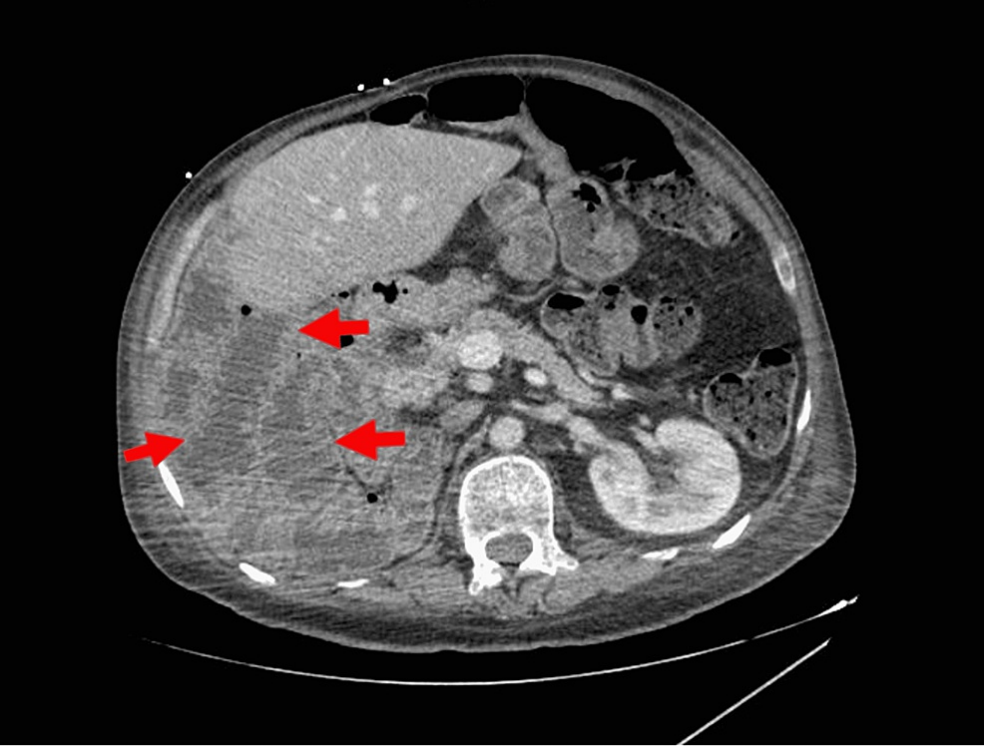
Transverse view CT of the abdomen. Red arrows demonstrating duodenal inflammation consistent with CD CD, celiac disease
